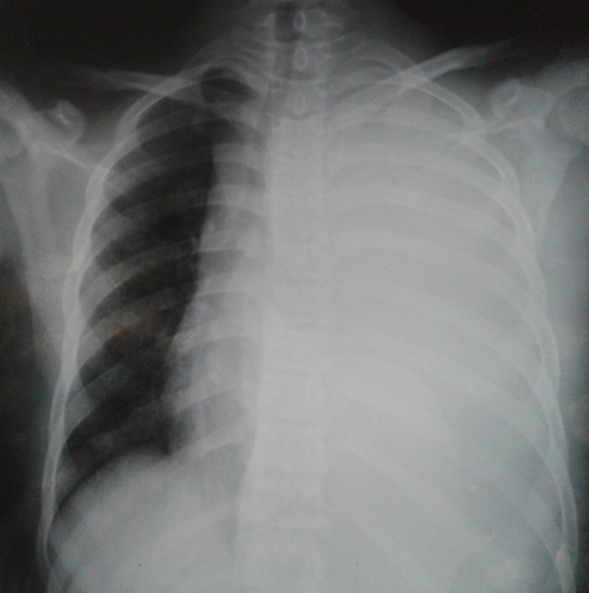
Radiographie thoracique montrant un hémithorax gauche opaque
